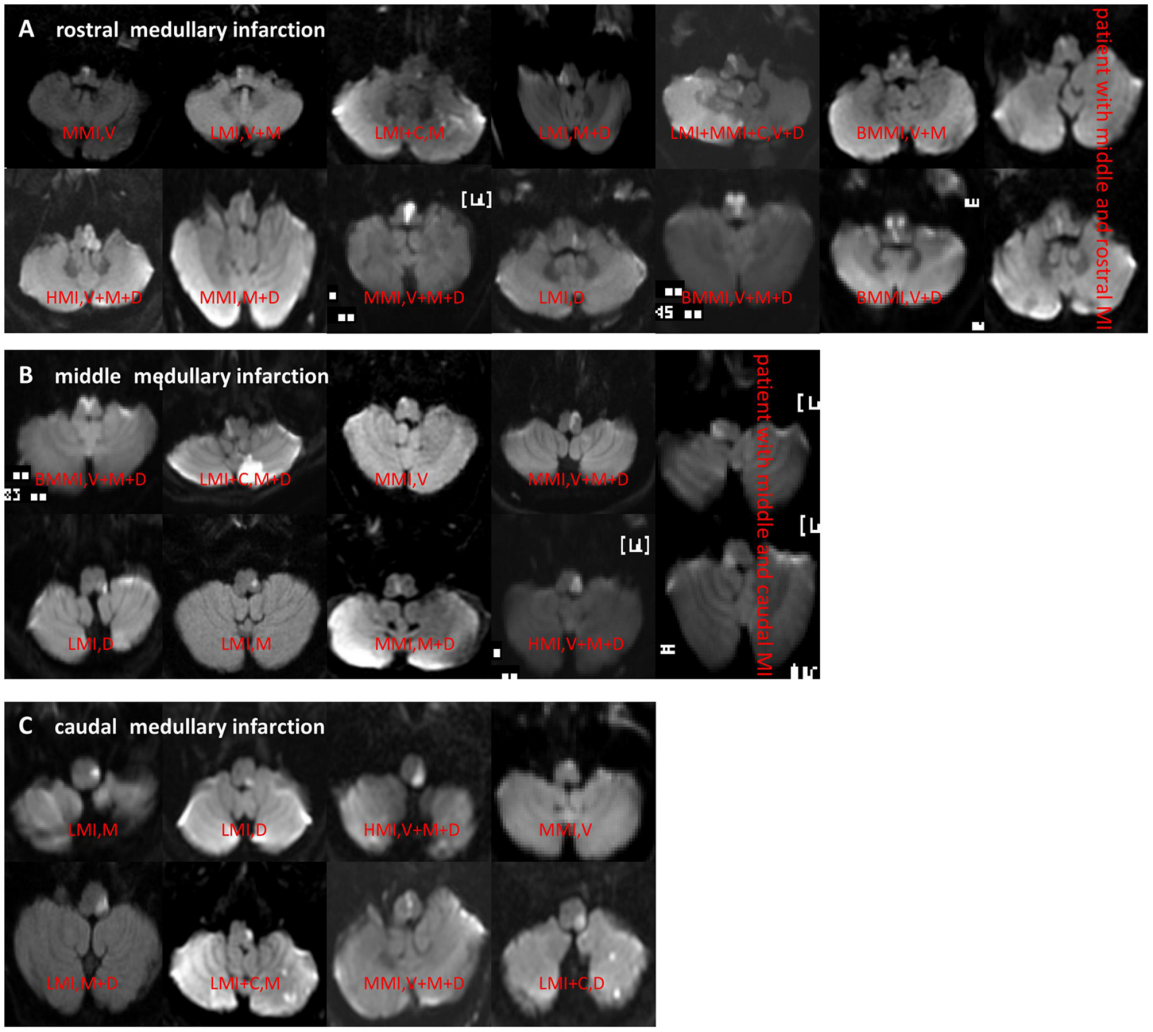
(A–C) DWI showed different types of rostral, middle, and caudal MI. MI, medullary infarction; LMI, lateral MI; MMI, medial MI; BMMI, bilateral MMI; LMI+C, LMI+cerebellar infarction; MMI+C, MMI+cerebellar infarction; HMI, hemimedullary infarction; V, ventral; M, middle; D, dorsal.
